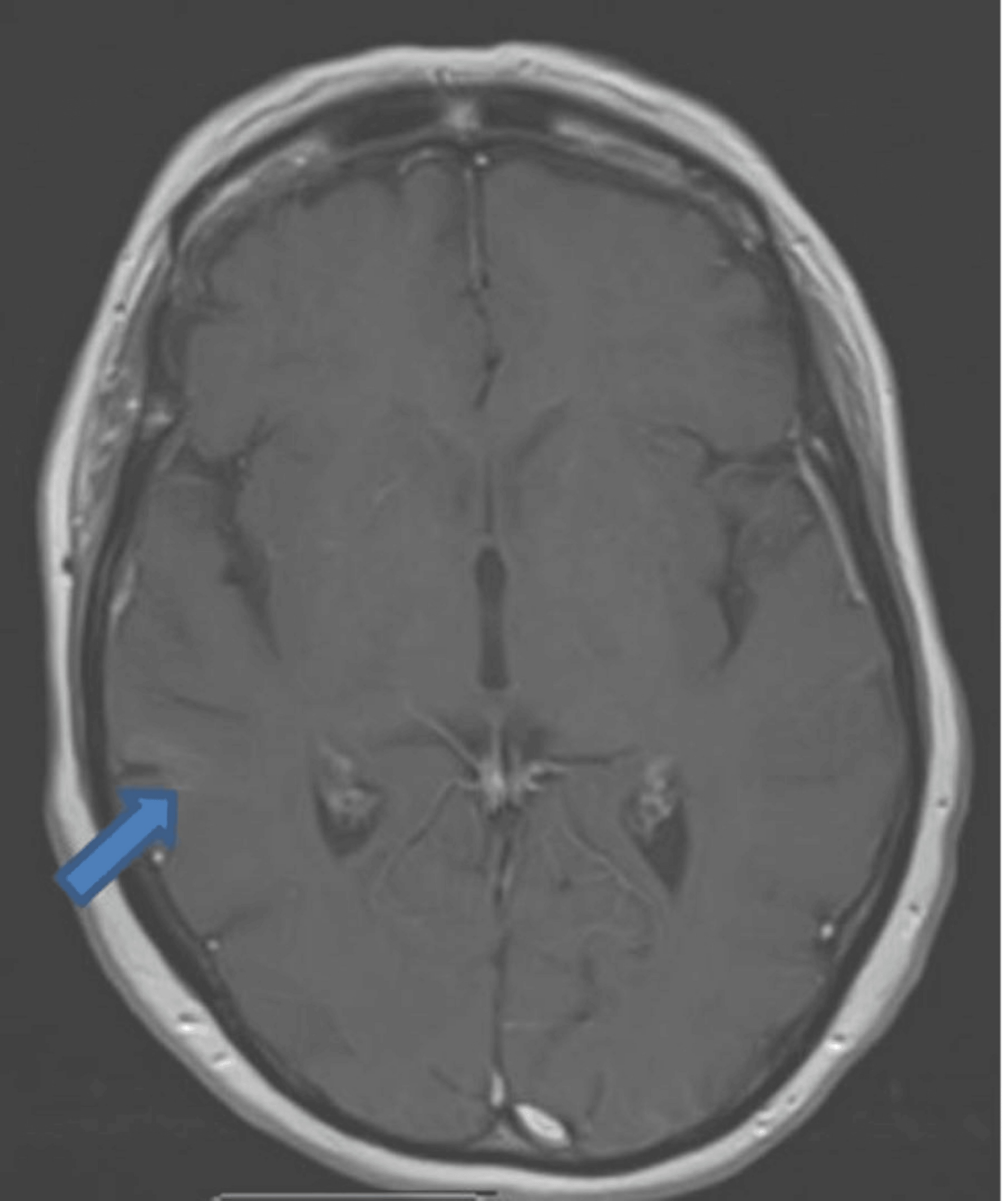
Head MRI without contrast (axial flair): faint diffusion restriction seen at the right parietal gyrus area (arrow)
